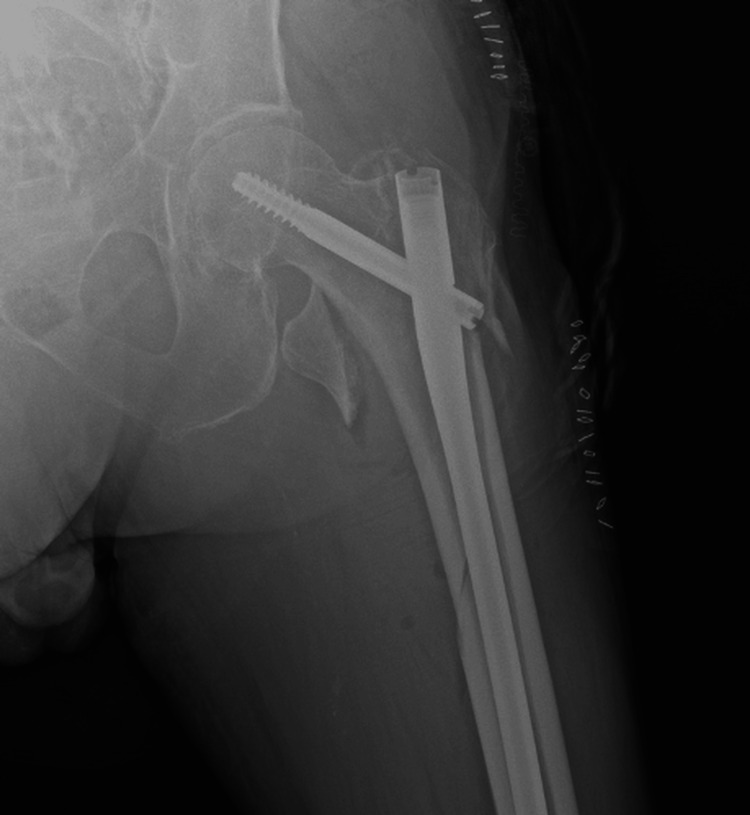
Postoperative X-ray Showing Intramedullary Gamma Nail Fixation of the left Subtrochanteric Femoral Fracture.
